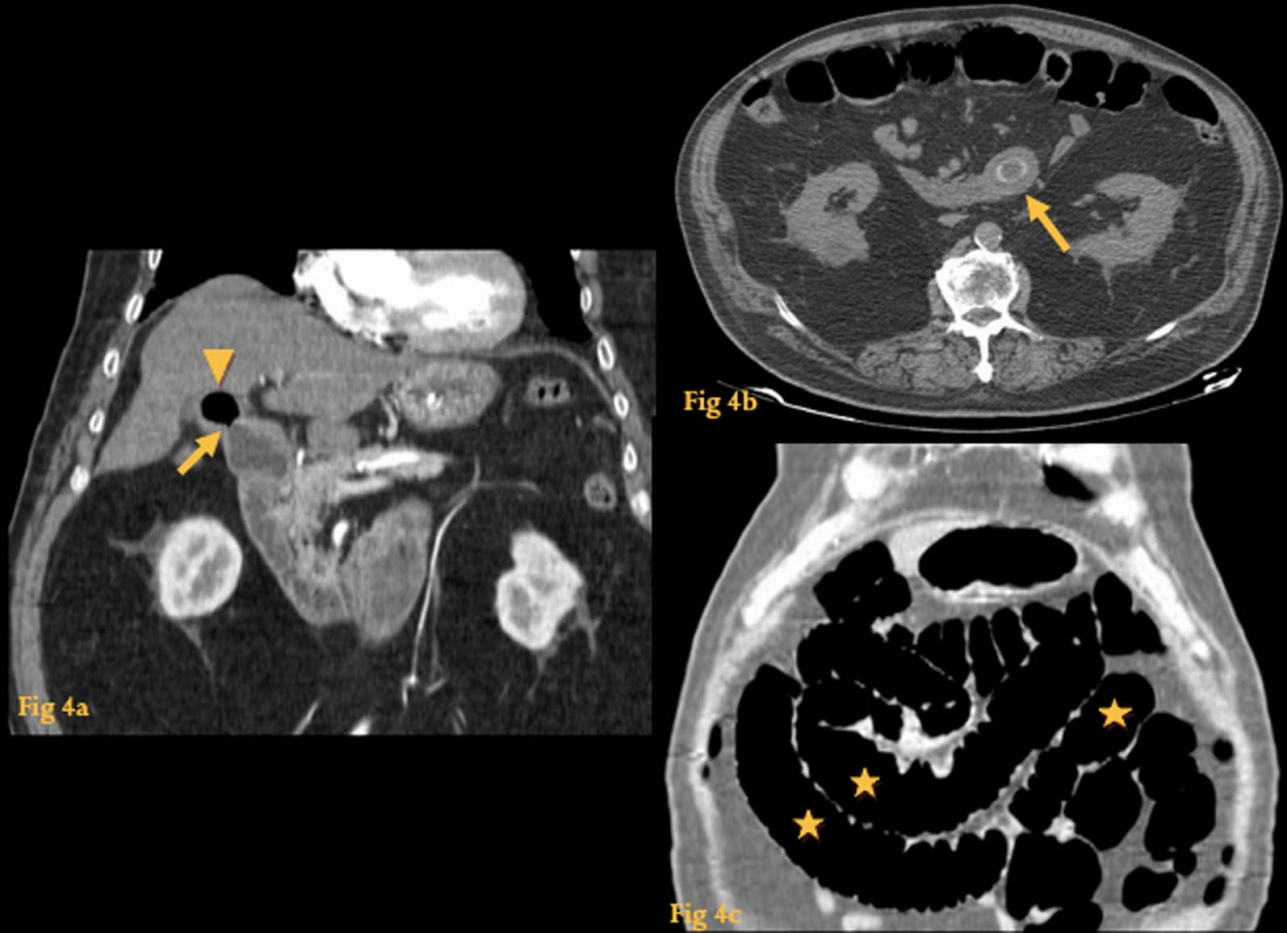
Biliary ileus. CT coronal reformation (a) shows air in the gallbladder (arrowhead) and communication between its lumen and D2—cholecystoduodenal fistula (arrow). Axial CT scans (b) show a 2 cm-wide elliptical gallstone impacted at the distal jejunum (arrow). Coronal reformation 9c) shows air-filled dilated small bowel loops (asterisk)
